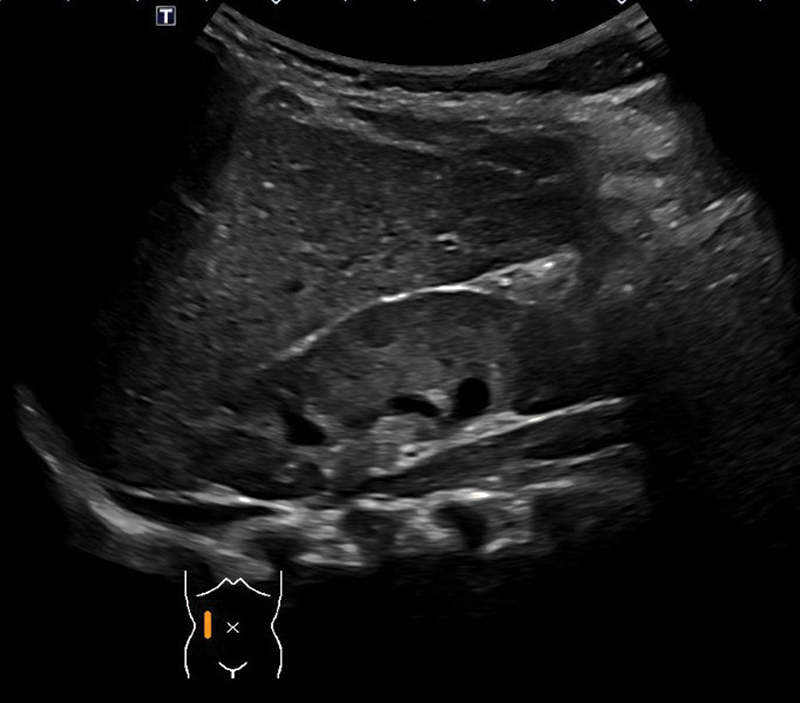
Ultrasound examination of right kidney at 1 year.
